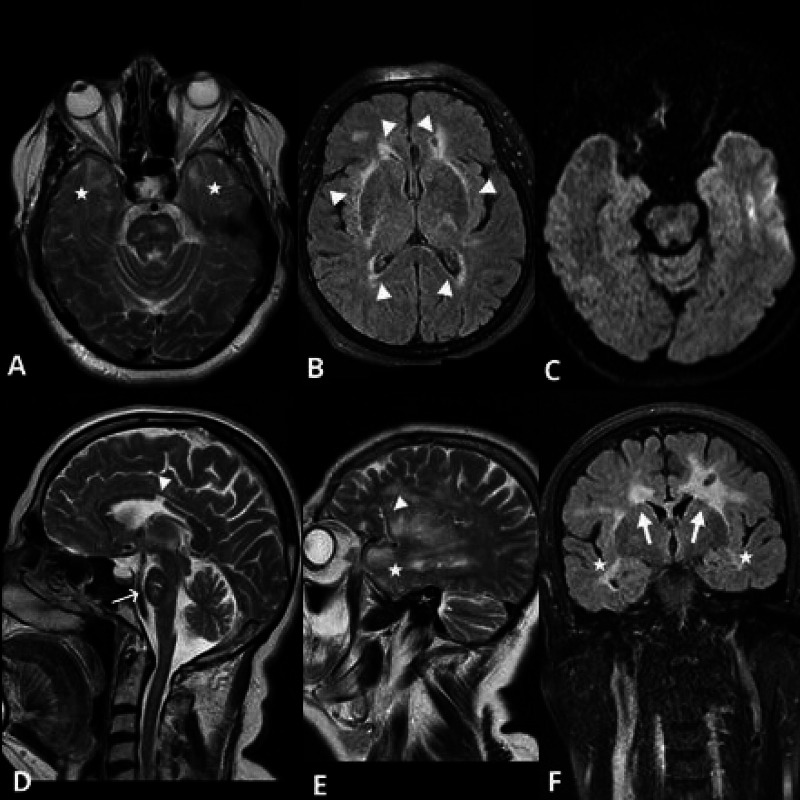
Axial T2 weighted and fluid-attenuated inversion recovery (FLAIR) images Axial T2 weighted and FLAIR images show high signal intensities on bilateral temporal subcortical white matter (asterisks, images A and C). On image B, involvement of the external capsules, posterior limbs of the internal capsules and periventricular white matter (arrow heads) are demonstrated. On sagittal T2 weighted image (image D), we see corpus callosum lesion (arrow head), pontine lesion (thin arrow), and frontal juxtacortical lesions. Parasagittal T2 weighted image (image E) depicts frontal subcortical white matter involvement (thin arrow). There is band-like external capsule involvement (thick arrow) and frontal-temporal (asterisks) periventricular and subcortical white matter lesions appreciated on coronal FLAIR image (image F).
